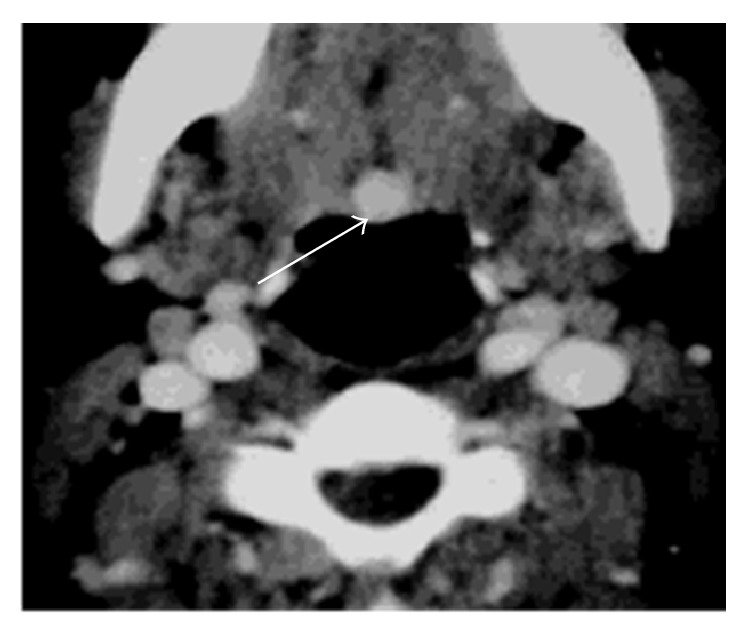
Axial CT: rounded lesion at the base of the tongue.
